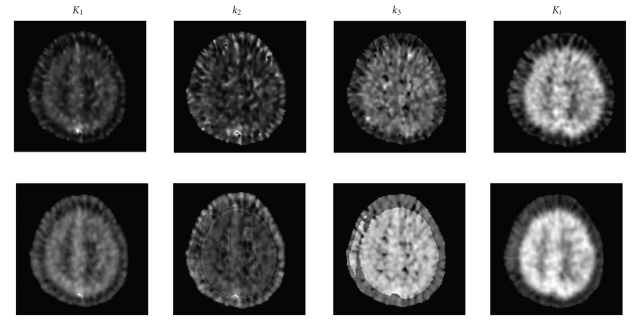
The parametric images of the 12th slice in clinical 3D FDG PET
